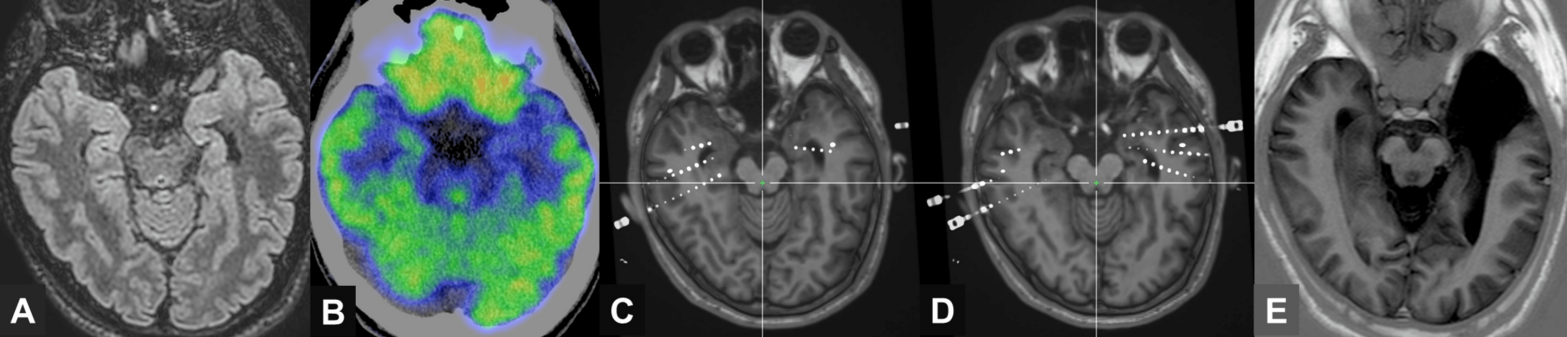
Multimodal imaging and electrode placement in a patient with bilateral temporal lobe epilepsy (A) Axial fluid-attenuated inversion recovery magnetic resonance imaging (MRI) showing bilateral hippocampal atrophy, which is more pronounced on the left side. (B) Interictal fluorodeoxyglucose-positron-emission tomography revealing hypometabolism in the left temporal lobe, including the mesial temporal structures. (C, D) Post-implantation axial MRI demonstrating the placement of stereoelectroencephalography electrodes targeting the hippocampus, entorhinal cortex, and lateral temporal cortex, bilaterally. (E) Postoperative MRI confirming the extent of left anterior temporal lobectomy, including hippocampal head resection.
